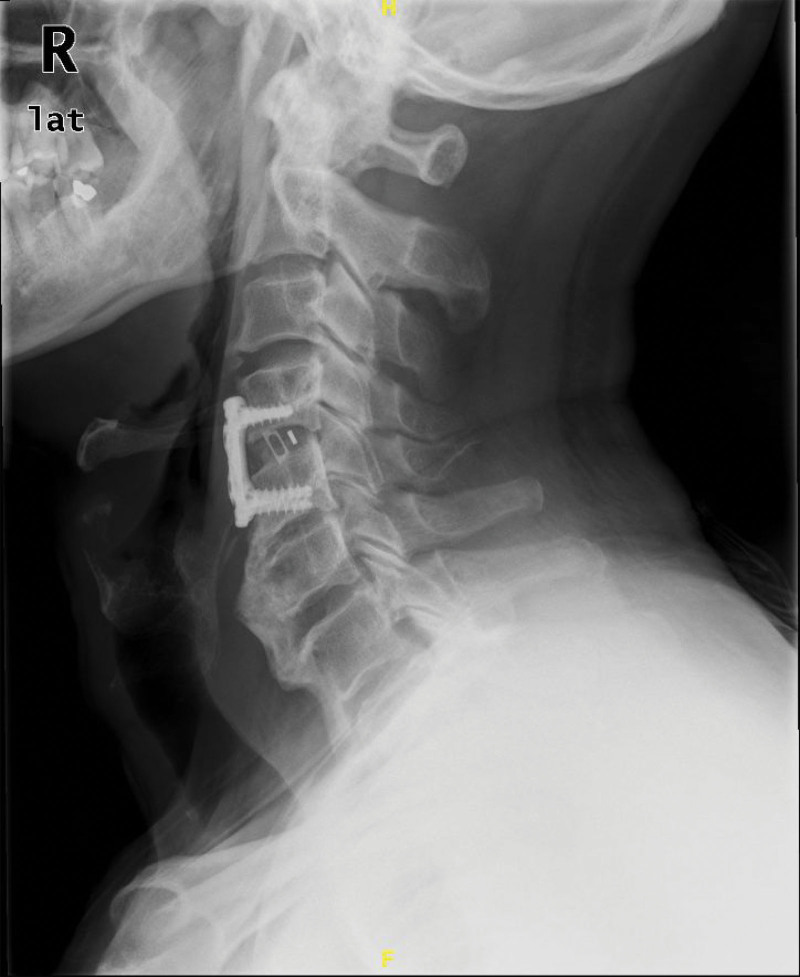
Lateral cervical X-ray at 1-year follow-up showing maintained alignment and instrumentation without significant osteophyte regrowth.
